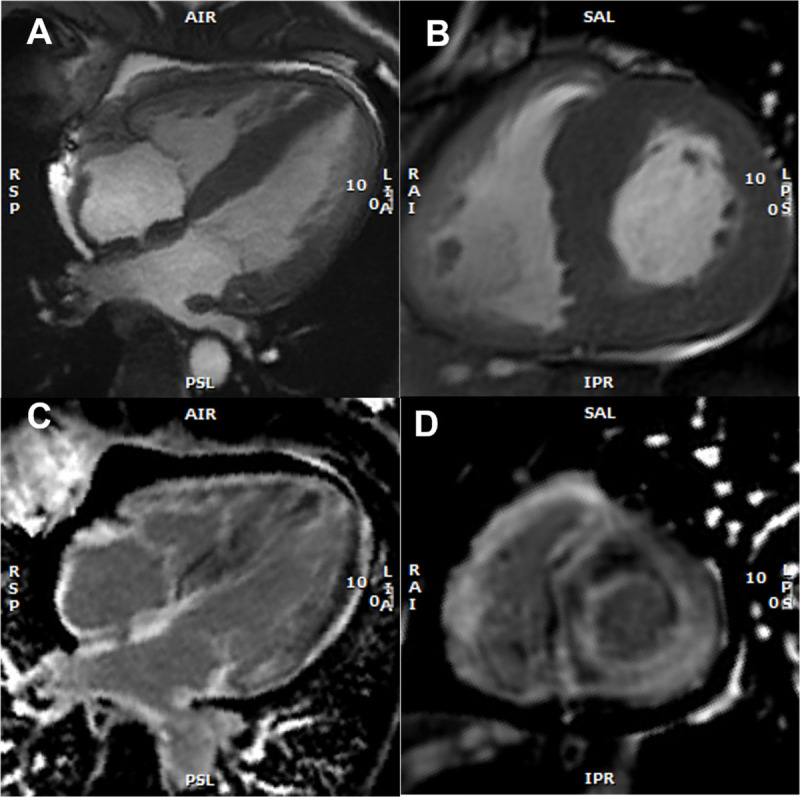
Cardiac magnetic resonance imaging findings with representative examples. (A) Cine: four-chamber view – *SSFP (steady-state free precession)* acquisition, depicting asymmetric left ventricular (LV) hypertrophy, inter-atrial septum thickening, and mild pericardial effusion (B) Short axis view – SSFP, asymmetric LV hypertrophy (C) Late gadolinium enhancement (LGE) at the 4-chamber view with subendocardial LGE at the left ventricle, atrial Wall, and inter-atrial septum (D) Short axis view, showing subendocardial and subepicardial LGE at the left ventricle and also at the right ventricle. (Images courtesy of Lusíadas Hospital, Lisboa, Portugal).
